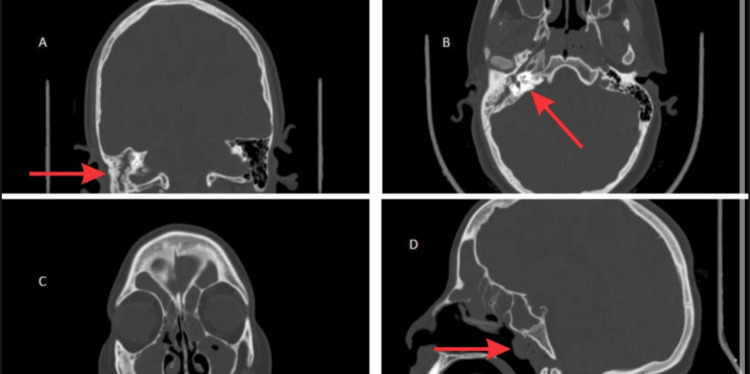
Non-contrast computerized tomography. Multiplanar bone window CT images show otomastoiditis (A), right petro-mastoiditis (B), adenoid hypertrophy (B,D), and gross pansinusitis (C,D).
